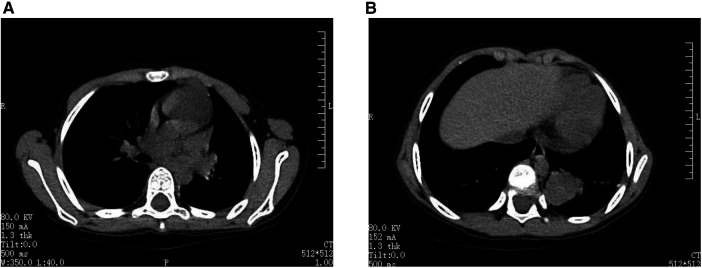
Chest CT of the patient. (A) Preoperative examination before bronchoscopy. CT scan revealed occlusion of the left main bronchus. (B) An irregular soft tissue mass was observed in the lower left lung.
